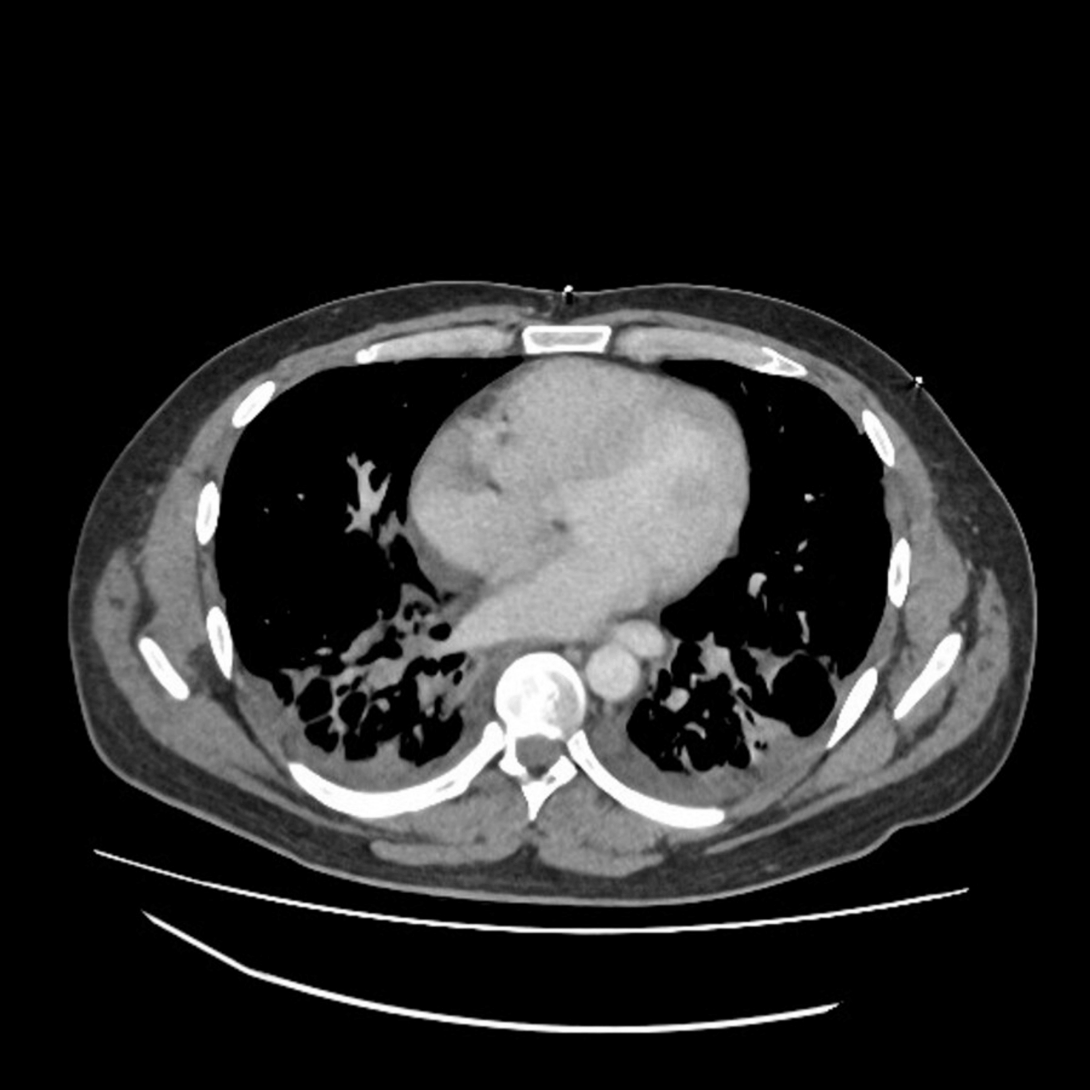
CT Chest showing bilateral symmetrical inflammatory changes in the lungs with small bilateral pleural effusions.
